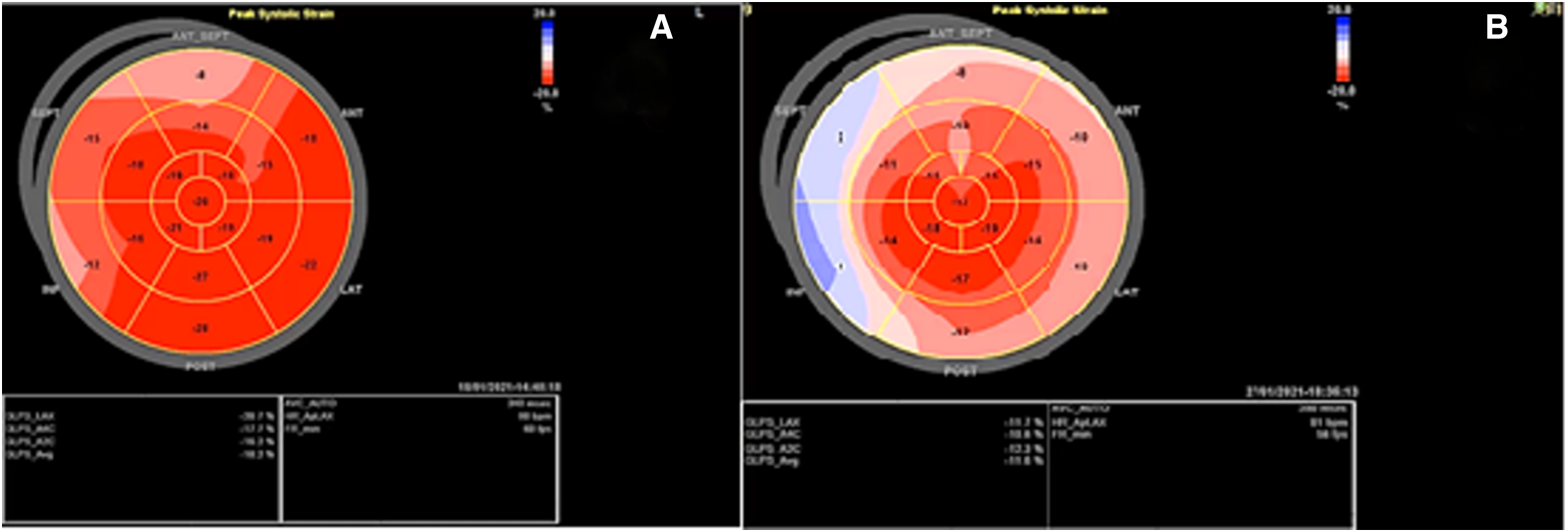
Speckle tracking echocardiography before and after the radiotherapy course: (A) Before radiotherapy, which showed a global longitudinal strain of −18.2% with myocardial performance impairment in the bases of inferior, septal, and anteroseptal walls a week. (B) After radiotherapy, which showed GLS: −11.2% with evidence of severe myocardial performance impairment accompanied by relative apical sparing.
